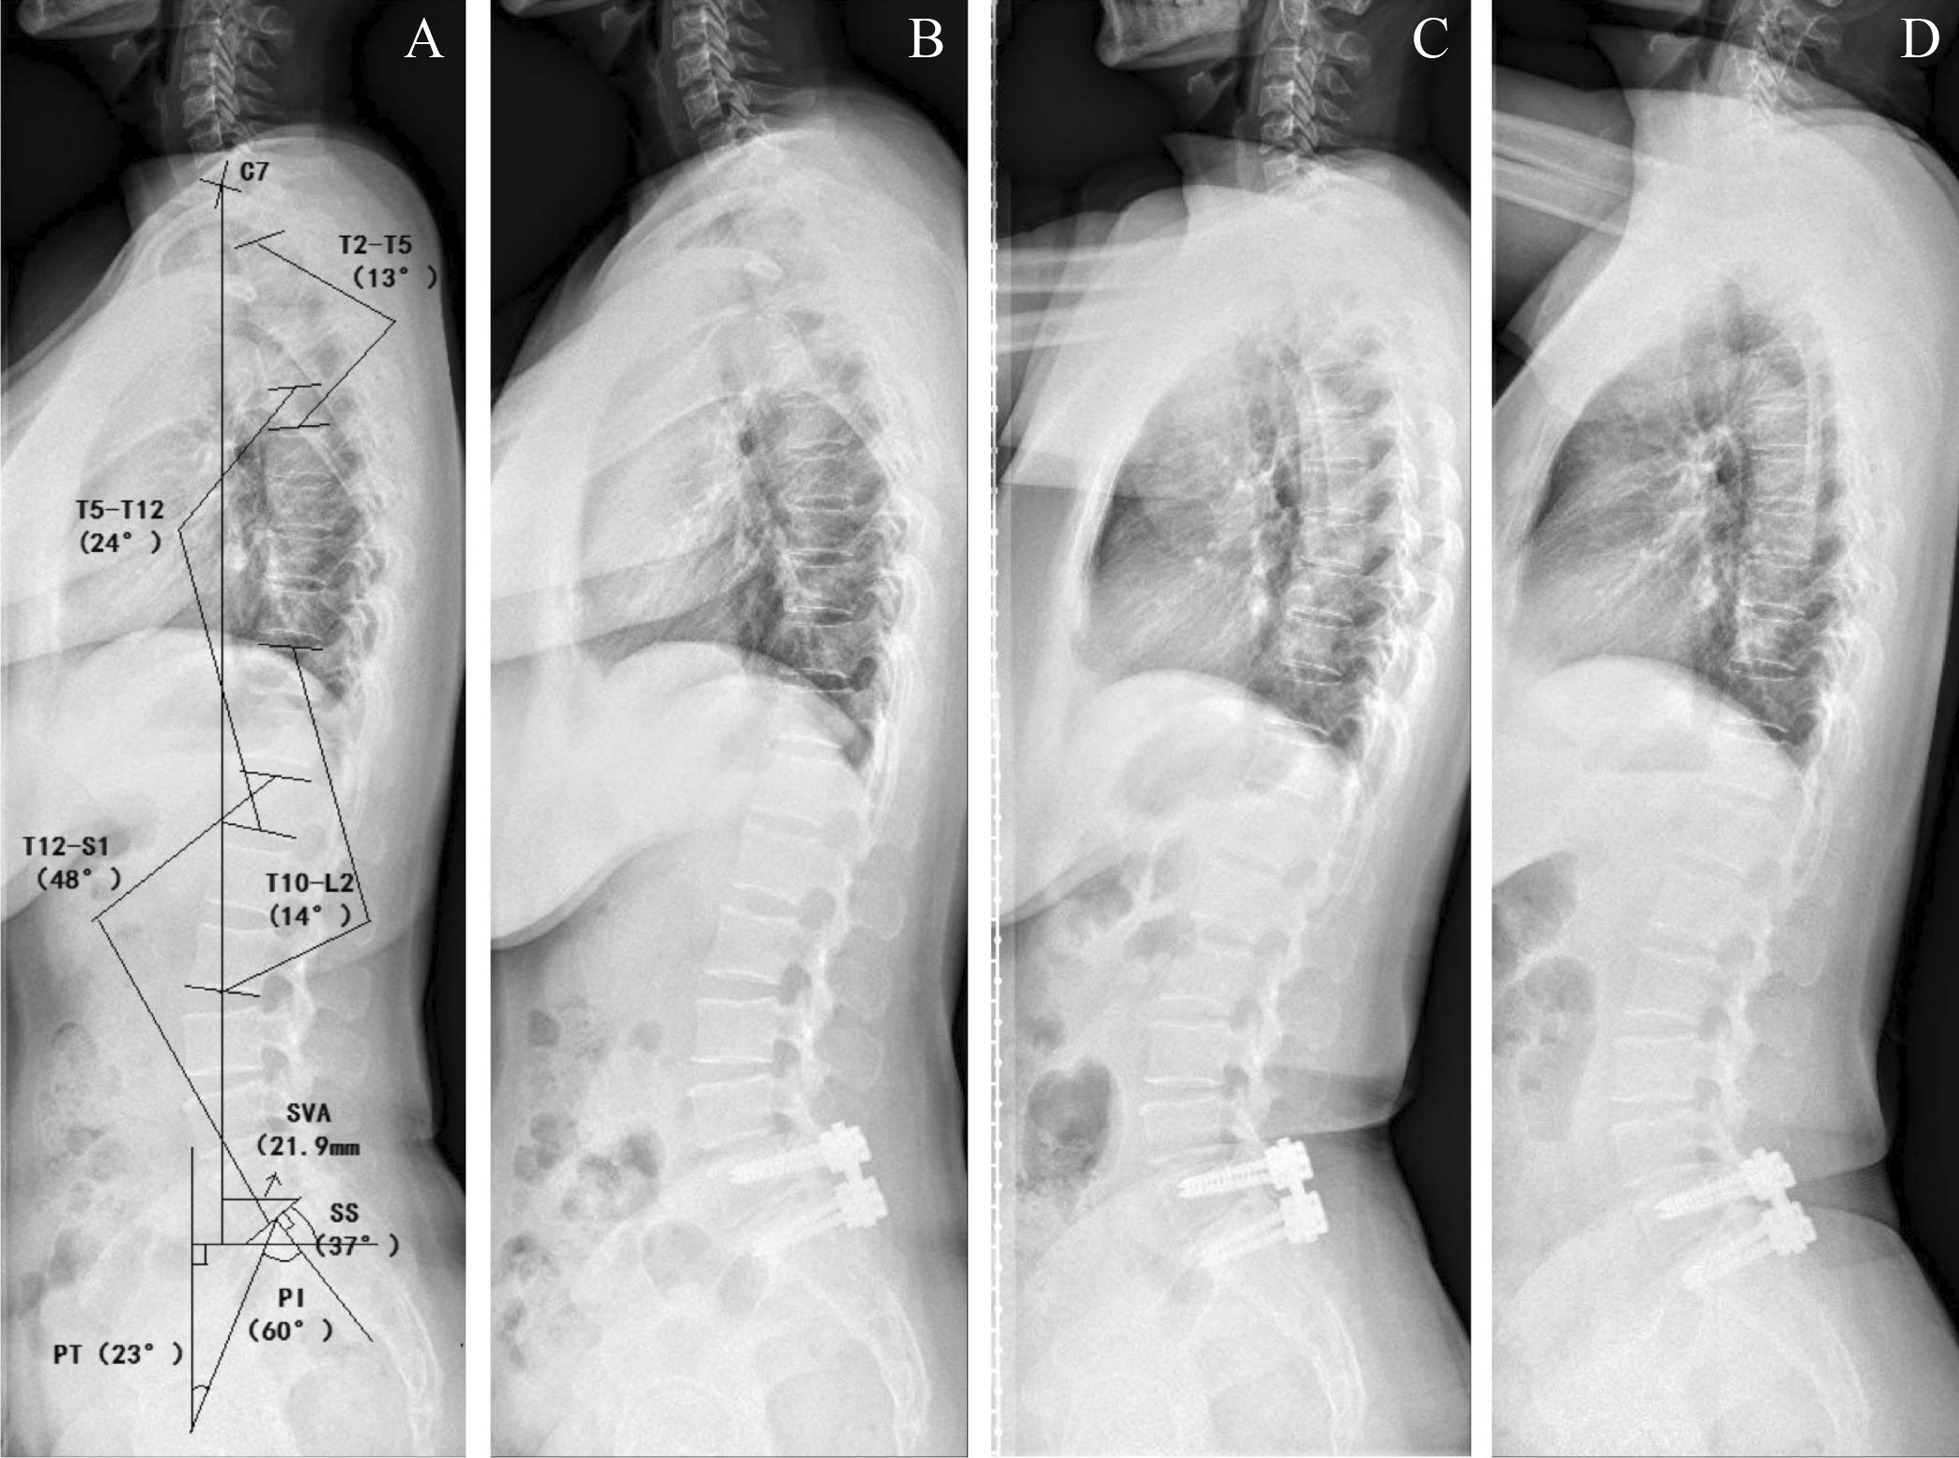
Lateral all-spine radiograph of baseline, 3-month, 6-month and 12-month follow-up. A Preoperative lateral all-spine radiograph. The method of measuring T2-T5, T5-T12, T10-L2, T12-S1, SS, PT, PI and SVA is shown in picture. B 3-month lateral all-spine radiograph. C 6-month lateral all-spine radiograph. D 12-month lateral all-spine radiograph
